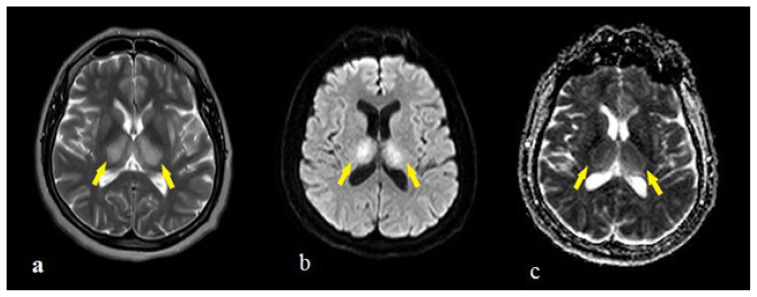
MRI appearences of a 54 years old female patient show T2 hyperintensity with expansion (a) and diffusion restriction (b,c) in bilateral thalamus and slightly in bilateral putamen (arrows).
